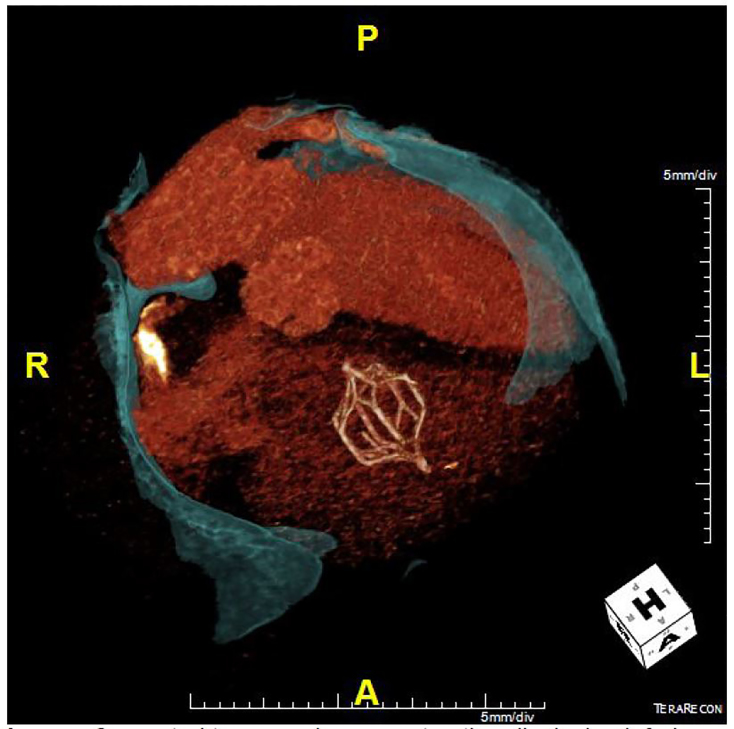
Computed tomography reconstruction displaying inferior vena cava filter lodged in right ventricle
